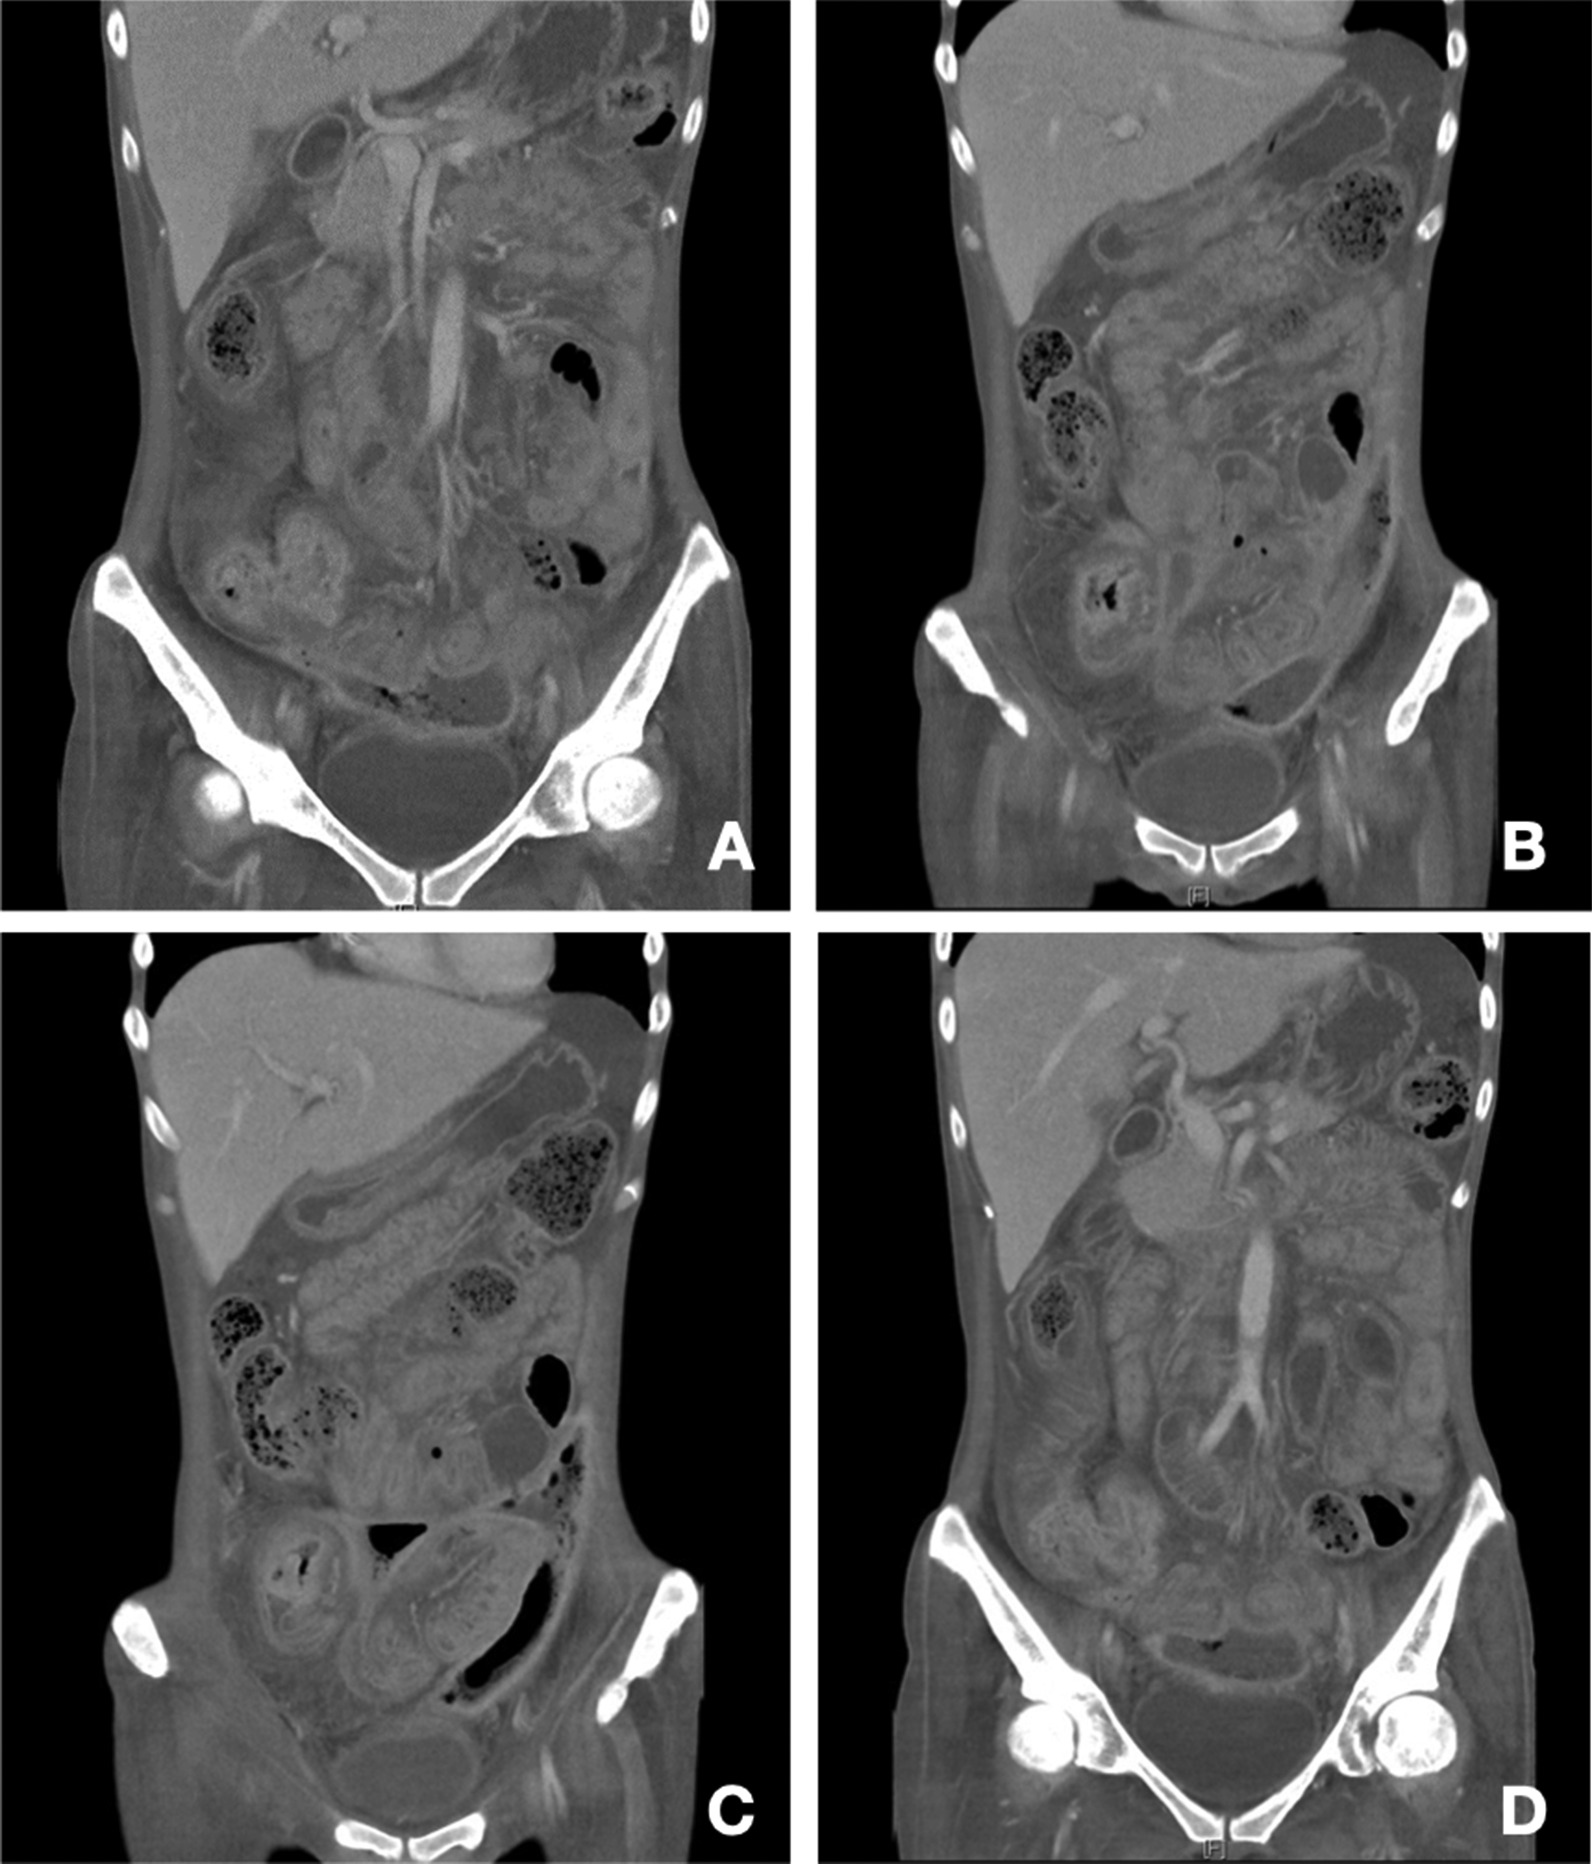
Computed tomography of the abdomen at diagnosis. A Wall thickening over the ascending colon, cecum and terminal ileum. B Suspicious terminal ileal perforation tract with a thick enhanced wall. C, D Irregular shaped fluid and gas collection with a thick, enhanced wall, extending from the upper to lower peritoneal cavity and cul-de-sac
